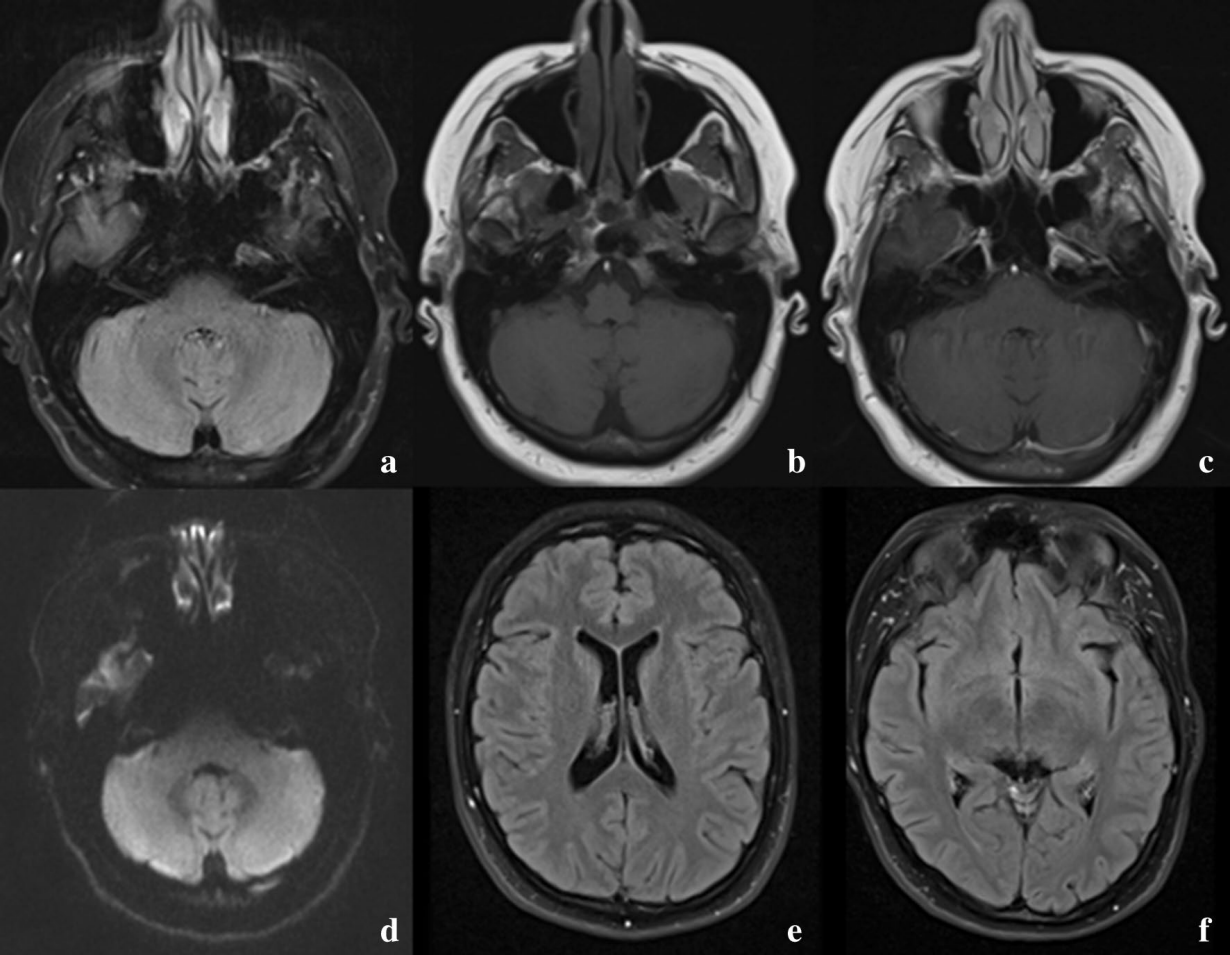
Follow-up magnetic resonance imaging (MRI) scan. From left to right, up to down: a FLAIR sequence, showing isointense cerebellar hemispheres. b T1 sequence, showing isointense cerebellar hemispheres. c T1 sequence with gadolinium, showing no contrast enhancement of the cerebellar hemispheres. d Diffusion sequence, showing no restrictive diffusion of the cerebellar hemispheres. e FLAIR sequence, showing normalized ventricles. f FLAIR sequence, showing normalized temporal horns
